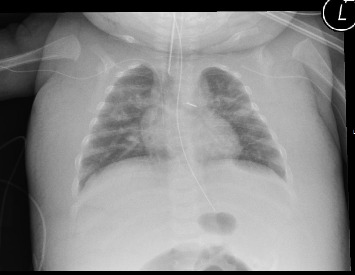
Chest X-ray of Patient B, showing remarkable subcutaneous edema and the presence of a central venous catheter in the left subclavian vein. The catheter on the left was removed after this X-ray confirmed the correct position of the right subclavian vein catheter.
